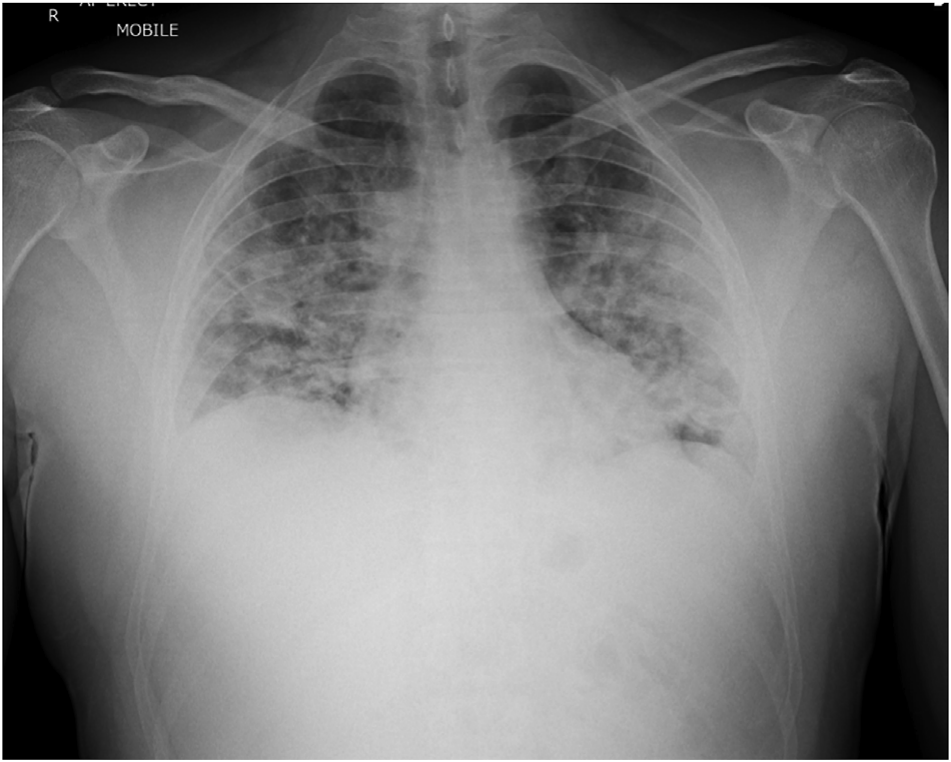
Chest radiograph of 38 year-old male, five days progressed from Fig. 1. Patient requiring increased oxygen supplementation and displaying increased work of breathing. Radiograph shows progression of bilateral infiltrates.
